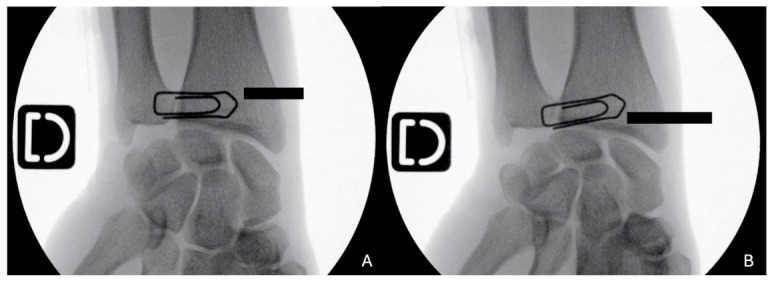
Radiographic images of the wrist after dynamic maneuvers; (A) Ulnar inclination at 30°, (B) Clenched fist pen incidence.
